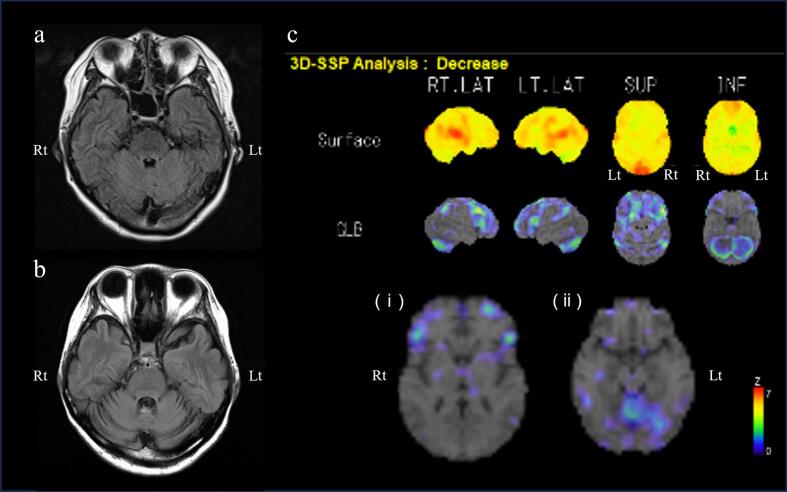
Temporal changes in brain magnetic resonance and perfusion images before surgery. (a) Transverse slices of fluid-attenuated inversion recovery (FLAIR) images during the acute phase of hyperthermia. (b) Transverse FLAIR images taken at nine months after onset, showing progressive atrophy of the bilateral superior cerebellar peduncles and the cerebellum. (c) Three-dimensional stereotactic surface projection analyses of ^123^I-iodoamphetamine single-photon emission computed tomography images, scanned nine months after onset. Statistical parametric image of reduced regional perfusion shown in rainbow color (Z score) compared to the healthy individual database, showing marked hypoperfusion in the bilateral cerebellum and mild hypoperfusion in the bilateral frontal lobes, but no abnormalities in the (i) basal ganglia and (ii) brainstem in the transverse slice. Rt: right, Lt: left.
